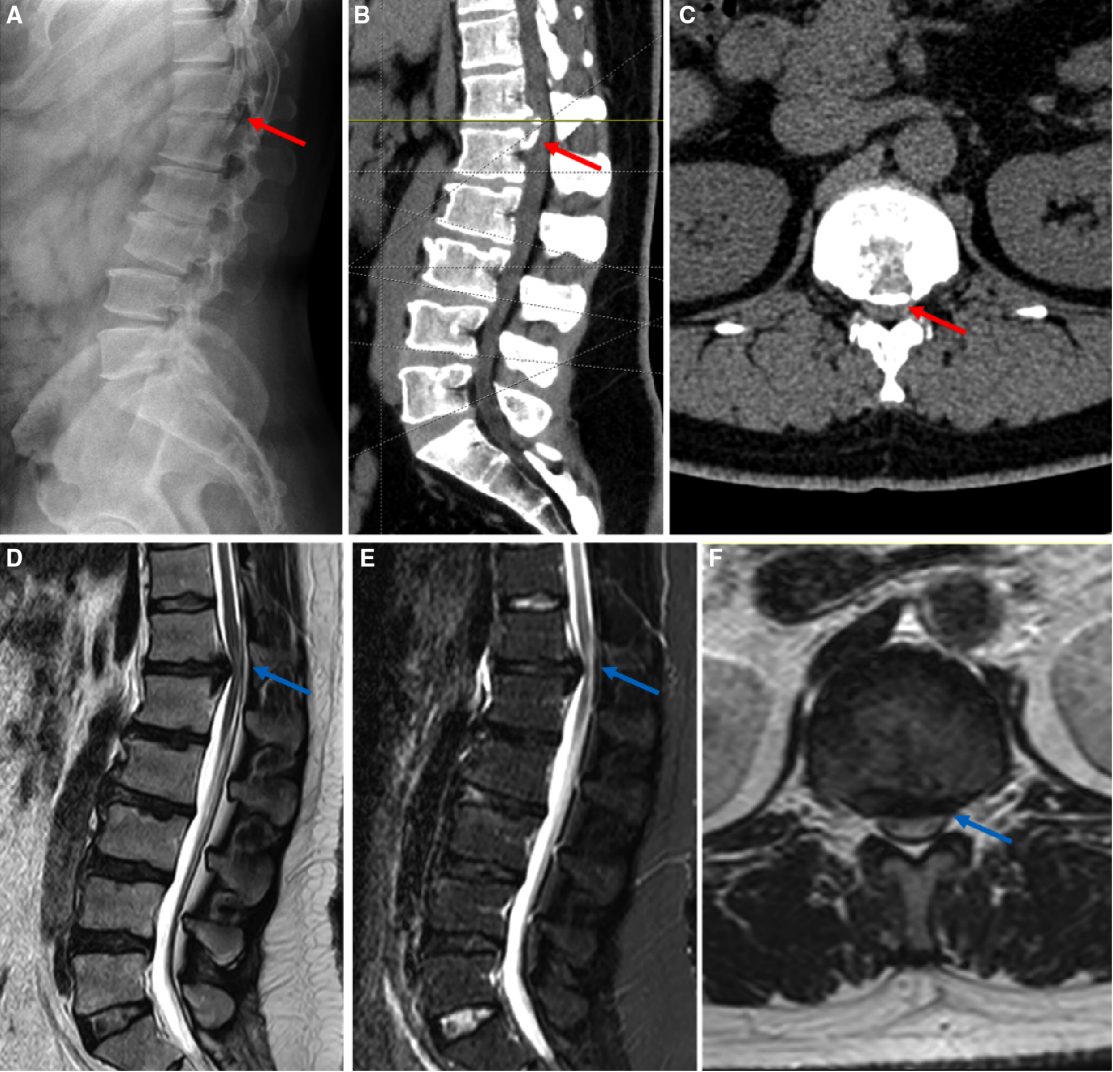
Preoperative imaging of the typical patient with disc herniation at the T12–L1 level. A 38-year male patient diagnosed with TLDH with calcification at the T12–L1 level underwent PETD under general anesthesia. (A–C) Preoperative x-ray and CT. The red arrow in (A–C) highlighted calcified herniated disc and ossified posterior longitudinal ligament. (D–F) Preoperative MRI. The blue arrows in (D–F) highlighted severe compression of spinal cord and the normal signal of cerebrospinal fluid cannot be seen. (A,B,D,E) sagittal view; (C,F) axial view; TLDH, thoracolumbar junction disc herniation; PETD, percutaneous endoscopic transforaminal discectomy; CT, computed tomography; MRI, magnetic resonance imaging.
